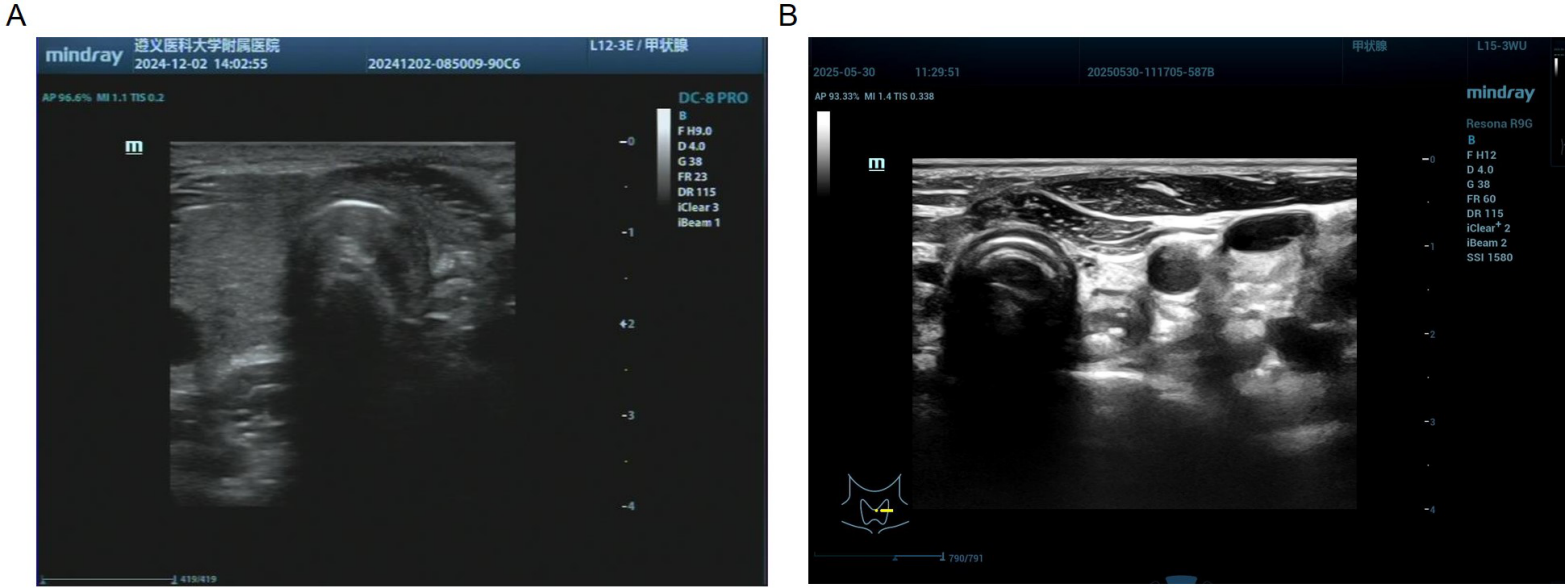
Results of Postoperative follow-up ultrasonography. (A) Ultrasonography performed on December 2, 2024, showed the left thyroid bed demonstrated absence of any residual glandular or nodular echoes. (B) Ultrasonography performed on May 30, 2025, still showed no recurrence.
